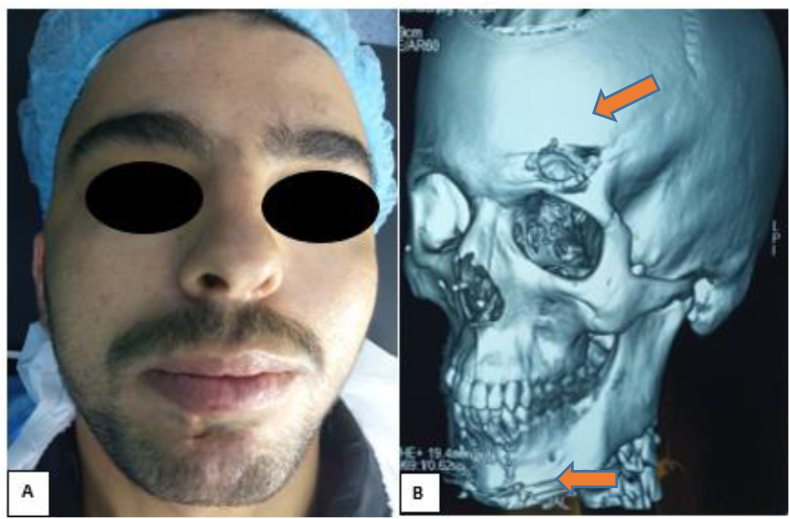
A: image of the patient showing scarred skin lesions of the projectile entry point at the left frontal and chin level, B: 3D reconstruction CT scan, showing foreign bodies of metallic appearance at the frontal and mandibular level.
